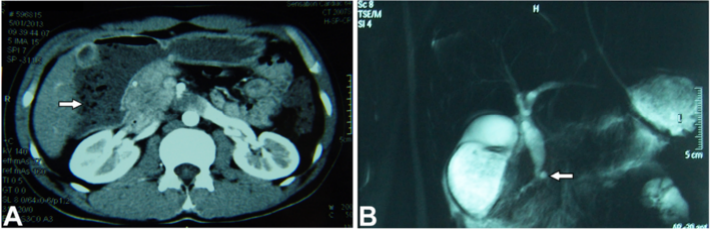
CT image and MRCP appearance. Computerized tomography scan showed the dilated descending part of duodenum (arrow) with stenosis at the distal end of dilated duodenum (A). Magnetic resonance cholangiopancreatography revealed fusiform dilatation of the common bile duct with high confluence of pancreaticobiliary ducts (arrow) with a common channel measured 24 mm in length (B).
